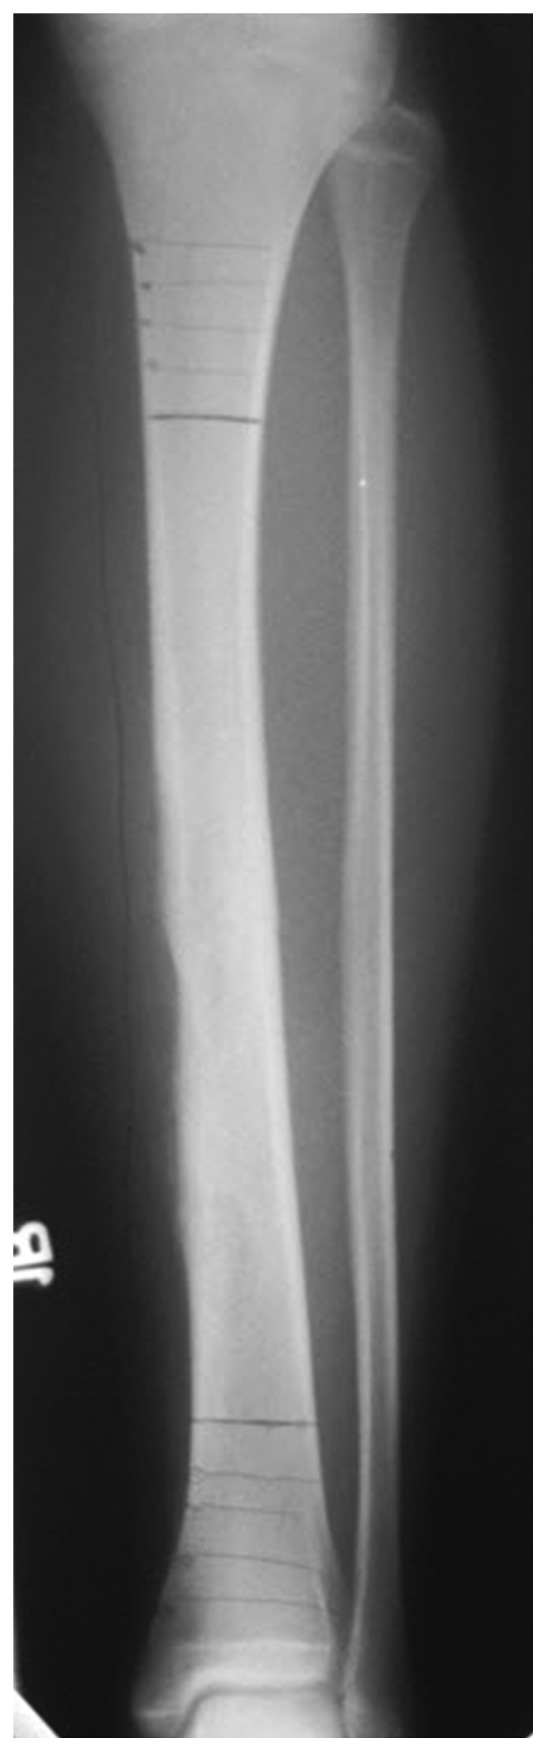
An anteroposterior radiograph of the right tibia and fibula obtained following curettage and bone grafting demonstrating a predominantly cortical-based expansile lesion involving the distal diaphysis consistent with adamantinoma.
